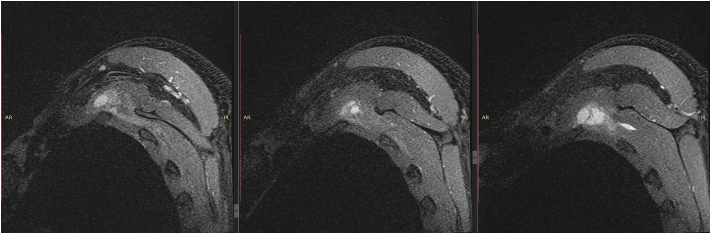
Preoperative MRI revealed bursitis in the left scapulothoracic joint.
